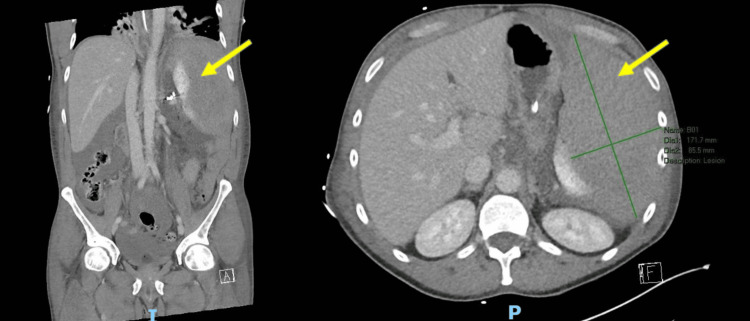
CT abdomen and pelvis demonstrating a persistent splenic hematoma (yellow arrow), suspected infarction of the pancreatic body and tail, and large-volume ascites consistent with hemoperitoneum. Coronal section on the left; axial section on the right. CT, computed tomography
